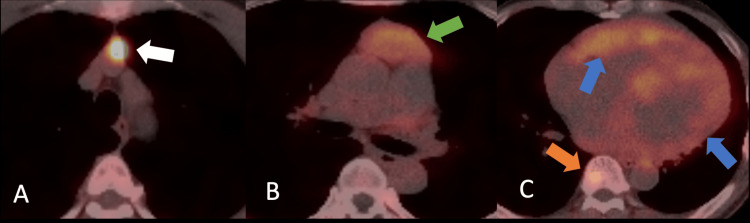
Initial staging DOTATATE PET/CT Images from the pretreatment gallium-68 DOTATATE PET/CT were analyzed, with standardized uptake values (SUV) reported as the maximum values normalized to lean body mass; the mean liver SUV was 4.9. (A) Axial image at the level of the thymus in the anterior mediastinum showing a mass (white arrow) with increased metabolic activity (SUV 17). (B) Slightly inferior, also in the anterior mediastinum, showing an additional mass (green arrow) with increased metabolic activity (SUV 4.3). These anterior mediastinal masses are consistent with neuroendocrine neoplasm. (C) Diffusely increased uptake in the left and right ventricles (blue arrows) (SUV 4.9). A focus of increased metabolic activity in the vertebral body of T9 (orange arrow) (SUV 2.3). PET/CT: positron emission tomography/computed tomography.
